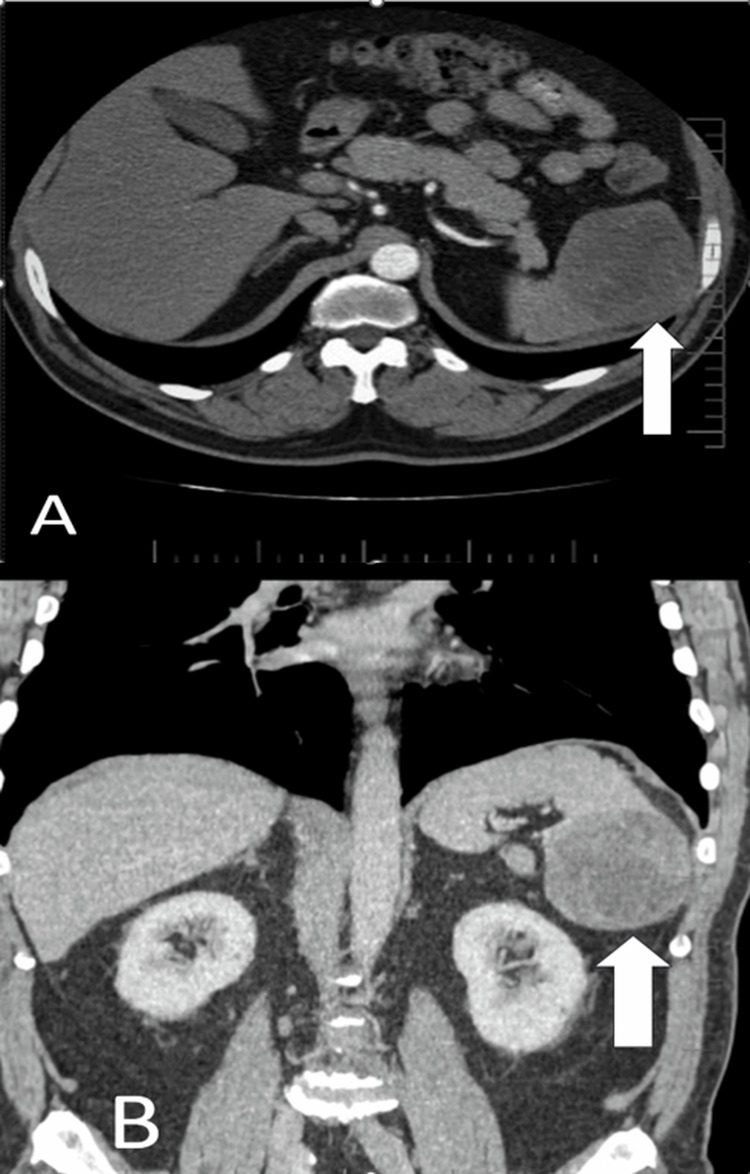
(A) Cross-section of abdominal organs: CT scan showing an oval, well-defined, hypodense lesion (~7 mm) in the lower pole of the spleen (arrow), with irregular peripheral soft tissue thickening and mild contrast enhancement. (B) Longitudinal section of abdominal organs: CT scan showing an oval, well-defined, hypodense lesion (~7 mm) in the lower pole of the spleen (arrow), with irregular peripheral soft tissue thickening and mild contrast enhancement.
